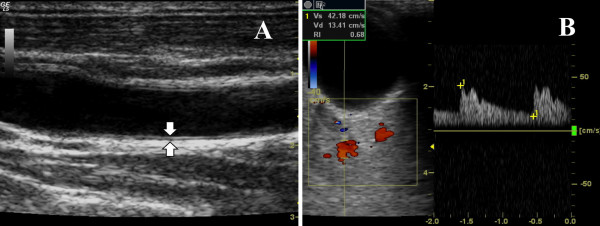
IMT and OARI measurement. (A) Common carotid intima-media thickness. Longitudinal scan obtained at 1 cm proximal to the origin of the carotid bulb shows the IMT (arrows). (B) Ophtalmic artery resistence index. Both measurements were evaluated by ultrasound frequency of 7.5 mHz.
